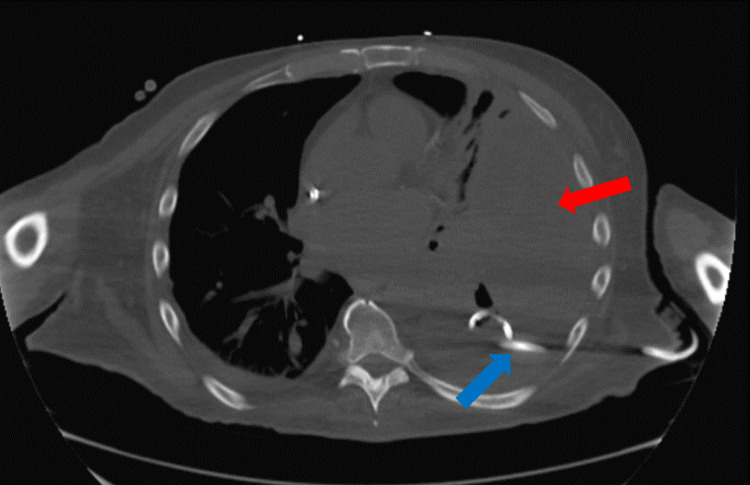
CT scan of the chest showing left-sided, large, and loculated effusion (red arrow) with chest tube in place (blue arrow). CT: computed tomography
